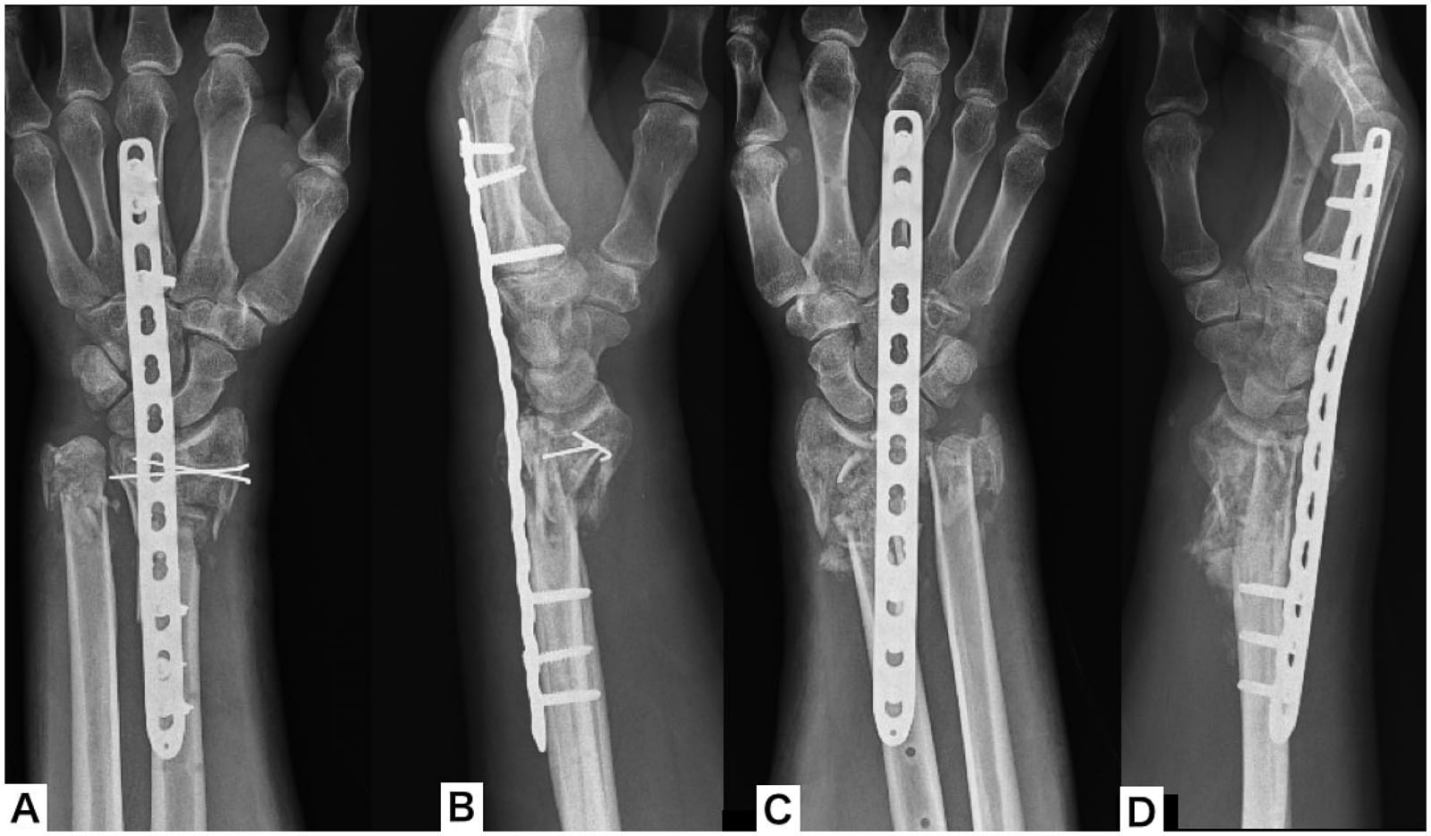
Plain x-ray of both wrists after distraction plating. (A,B) Plain x-ray of the left wrist; (C,D) plain x-ray of the right wrist.
